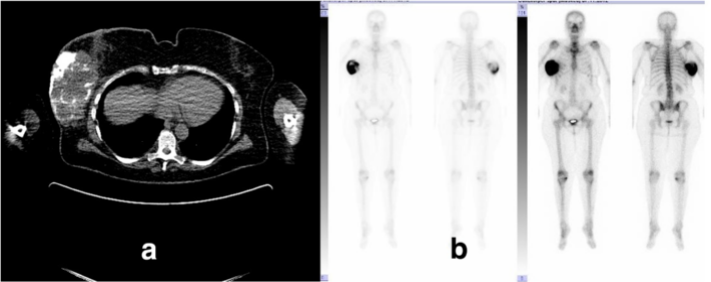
a Computed tomography scan of the chest demonstrates a calcifying tumor mass of the right breast. No other tumor in chest or elsewhere was seen. b Scintigraphy shows a huge tumor in the right breast with calcification areas. No others tumors at the bone site were seen
